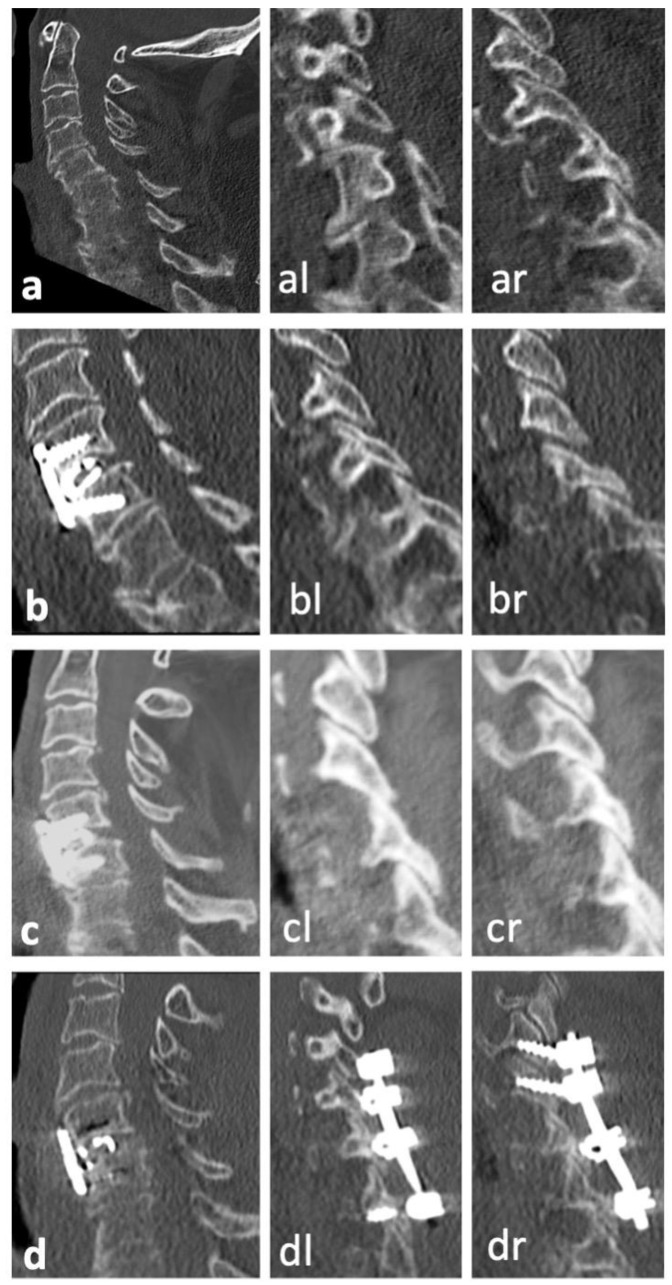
Representative CT images of patient no. 11 (Table 6). (a). Postinjury CT scan with midline sagittal reconstruction as well as perched left facet joint (al) and subluxation of right facet joint (ar) in parasagittal reconstructions. (b,bl,br). Corresponding CT reconstructions after anterior discectomy, intervertebral cage, and plate fixation C6/7 with adequate reduction in the anterior column and right facet joint (br) but persisting slight subluxation of the left facet joint (bl). (c,cl,cr). The patient presented with persisting neck pain five weeks post operation. CT reconstructions showed secondary dislocation with instability of the anterior column as well as increased subluxation of the left facet (cl). (d,dl,dr). Situation after posterior open reduction and additional posterior stabilization C4/5/6/7. While anatomical reposition of the anterior column was not achieved, stability was increased leading to pain relief and no further dislocation upon follow ups.
